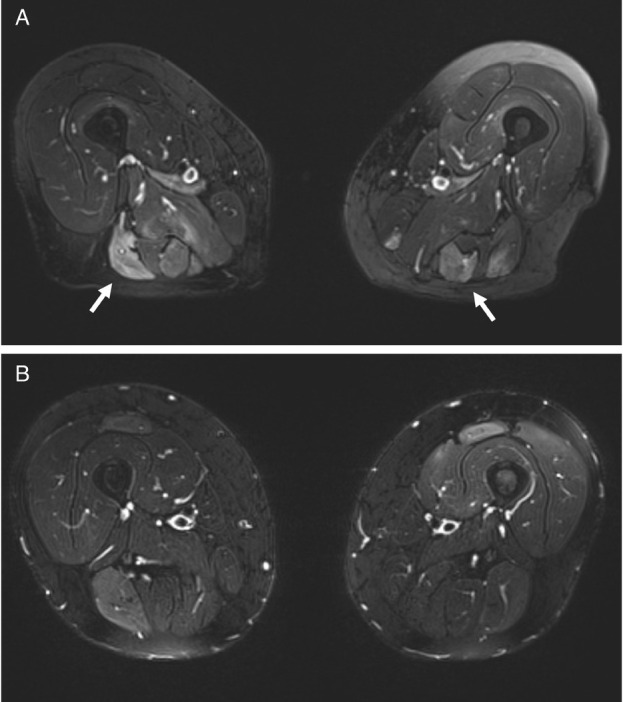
MRI scans of the thighs before and after rituximab therapy in Case 1. (A) Axial T2-weighted images of the upper thigh (December 2020) showing extensive areas of hyperintensity with muscle edema (white arrows). (B) Follow-up axial T2-weighted images (May 2022) of the same region demonstrate marked resolution of the previously observed edema.
